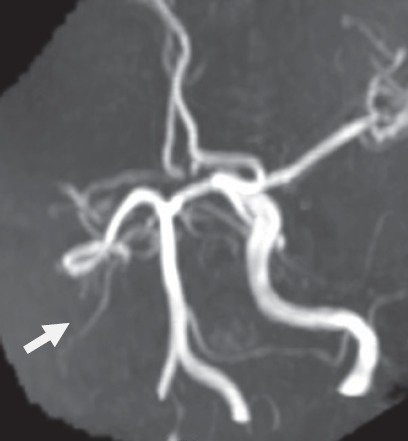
Angio-MRI no flow in the right ICA
